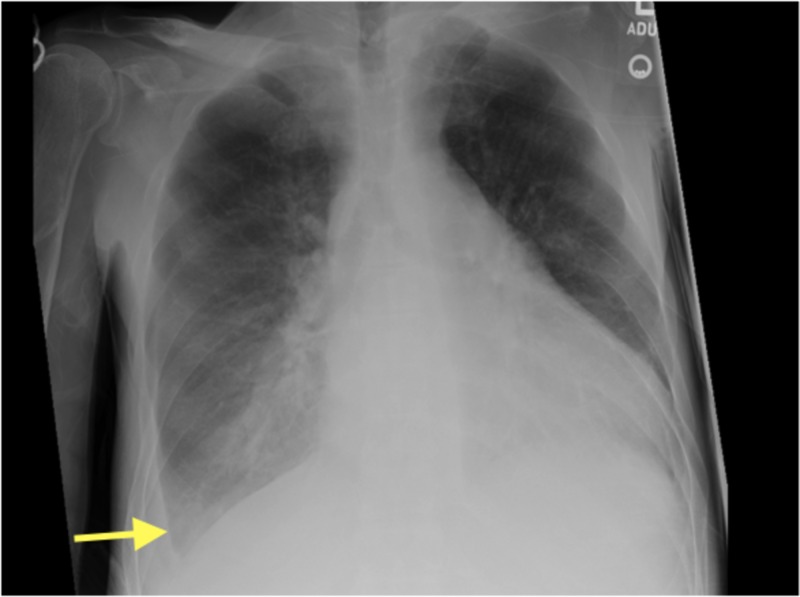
Chest X-Ray Arrow showing right pleural effusion
